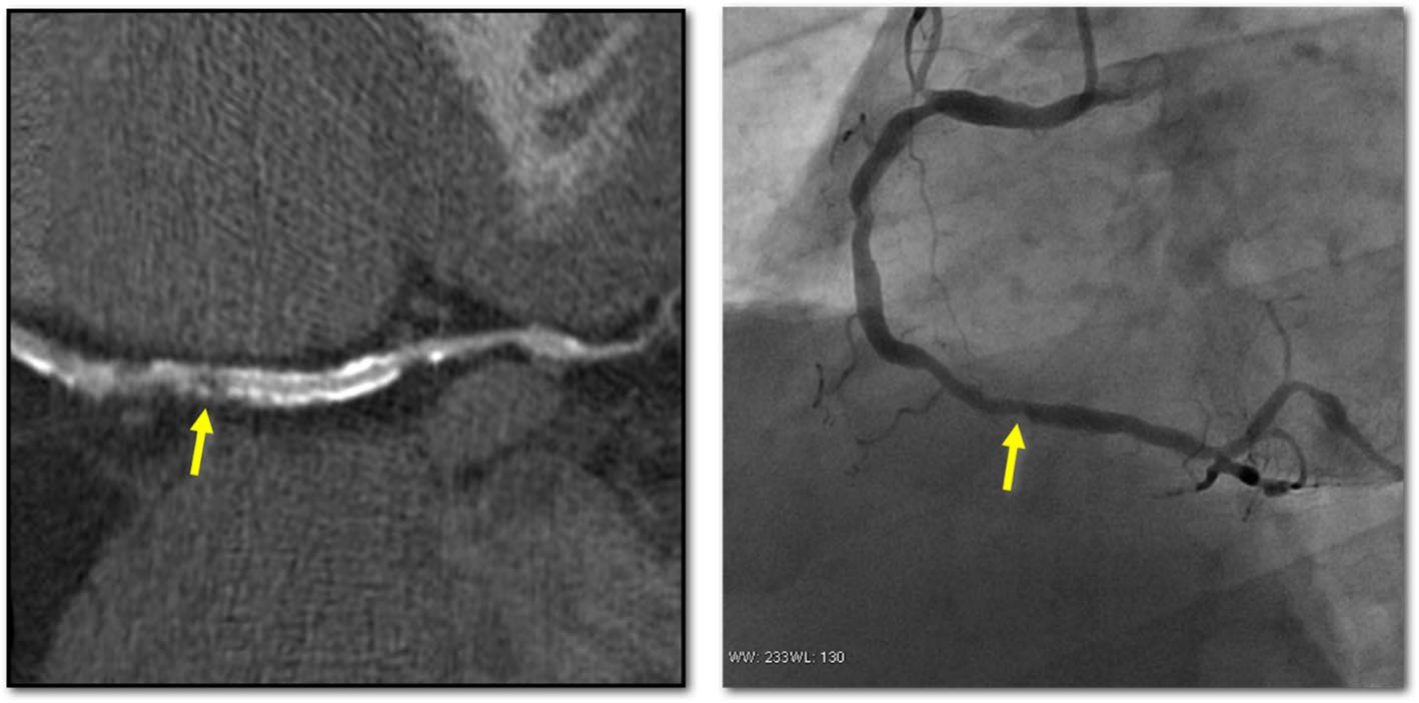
In this CT image, blooming causes overestimation of the severity of ISR in the proximal part of this bare metal stent (yellow arrow). ICA reveals the stenosis to be marginal
